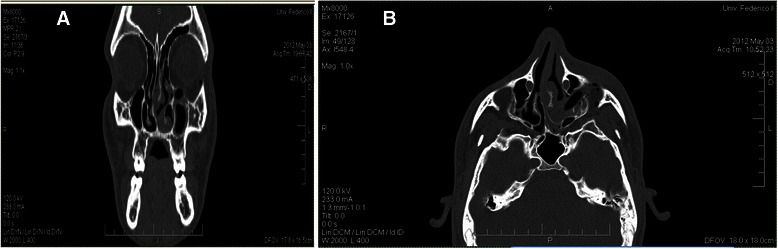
a–b Axial and coronal views of computed tomography images. A left orbital floor fracture and a foreign body localized in the medial portion of the left orbital floor, which extends upward to the left lower turbinate, can be observed
